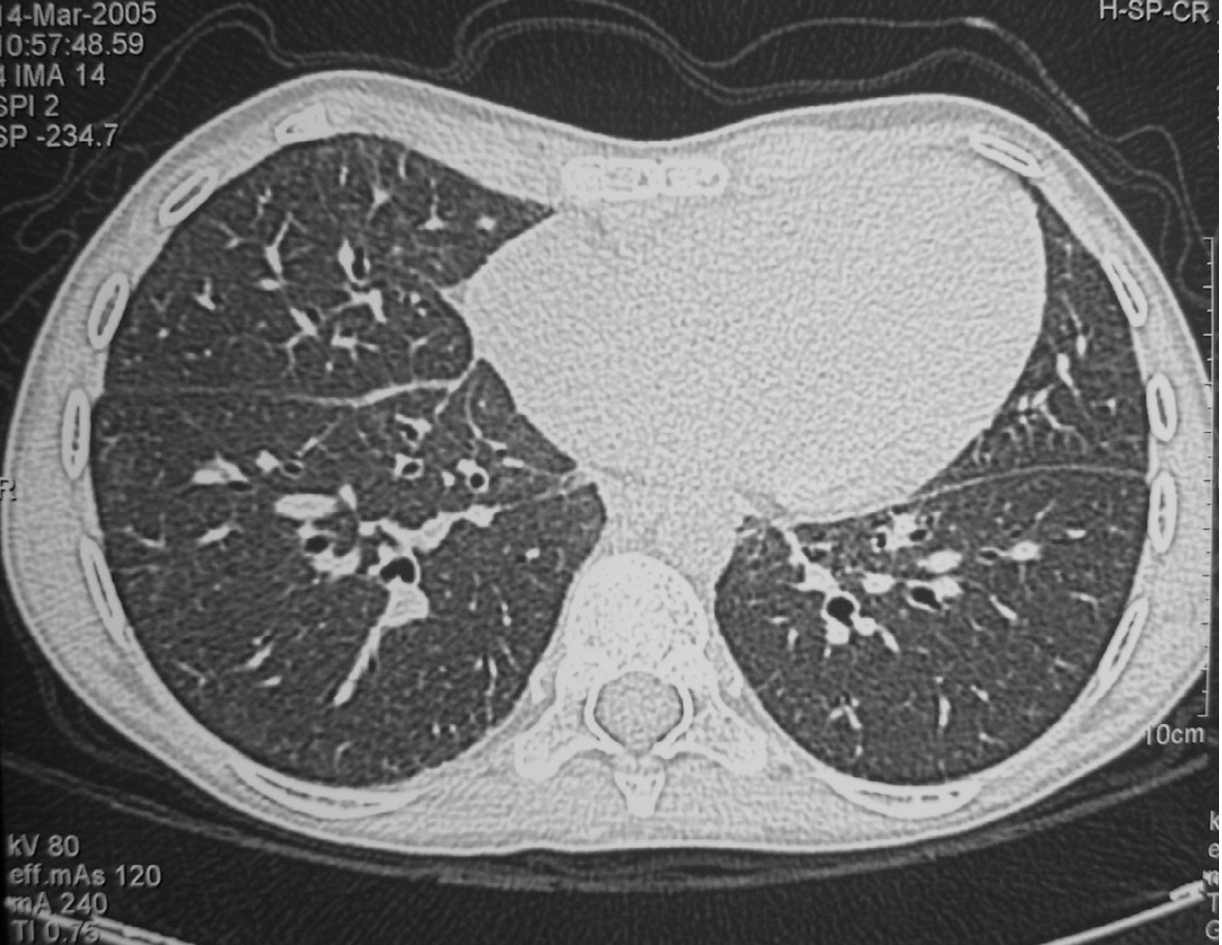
Chest HRCT performed 10 months after WLL. Areas of ground glass attenuation are no longer visible; only minimal interlobular septa thickening is still evident.
